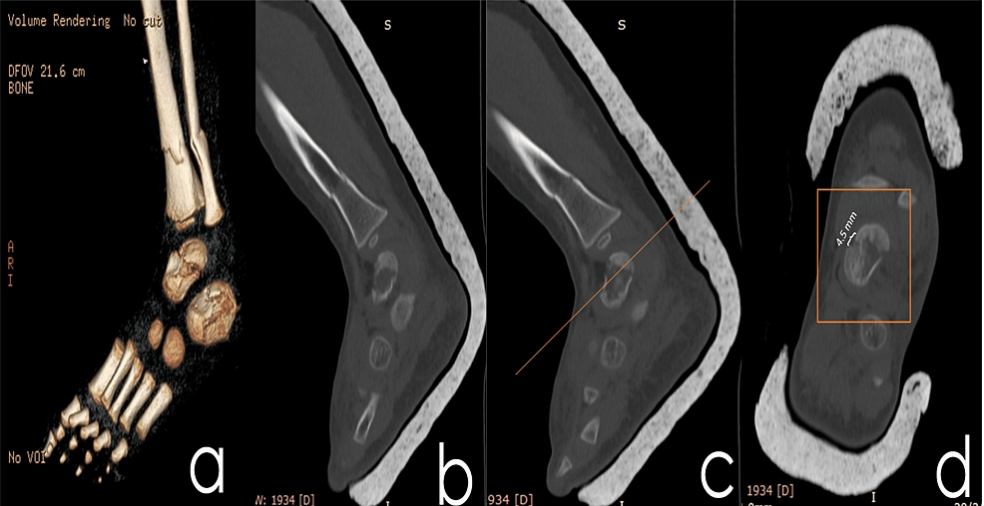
The multiple fractures of the case, as depicted at the CT, performed on the first day the patient presented at the emergency department (a) 3D reconstructive imaging; (b and c) sagittal views showing tibial, talus, and calcaneus fractures; and (d) axial view of the talus fracture showing 4.5 mm displacement in the talus fracture
